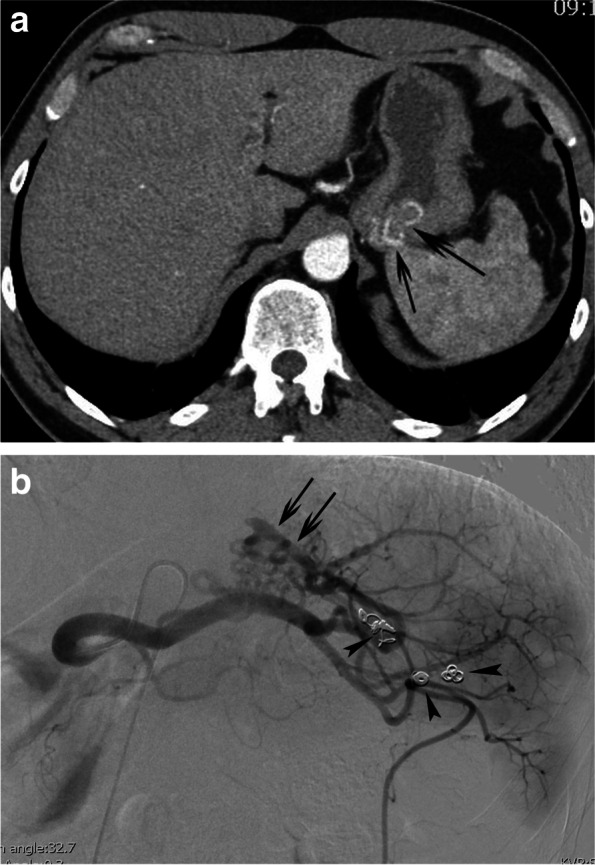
a Arterial-phase, contrast-enhanced CT reveals a cluster of enlarged arteries (black arrows) in the posterior portion of the gastric fundus. b Corresponding selective splenic artery angiography shows a cluster of hypertrophied arterial collaterals (black arrows), between the splenic artery main branch and the upper splenic pole segmental arteries
